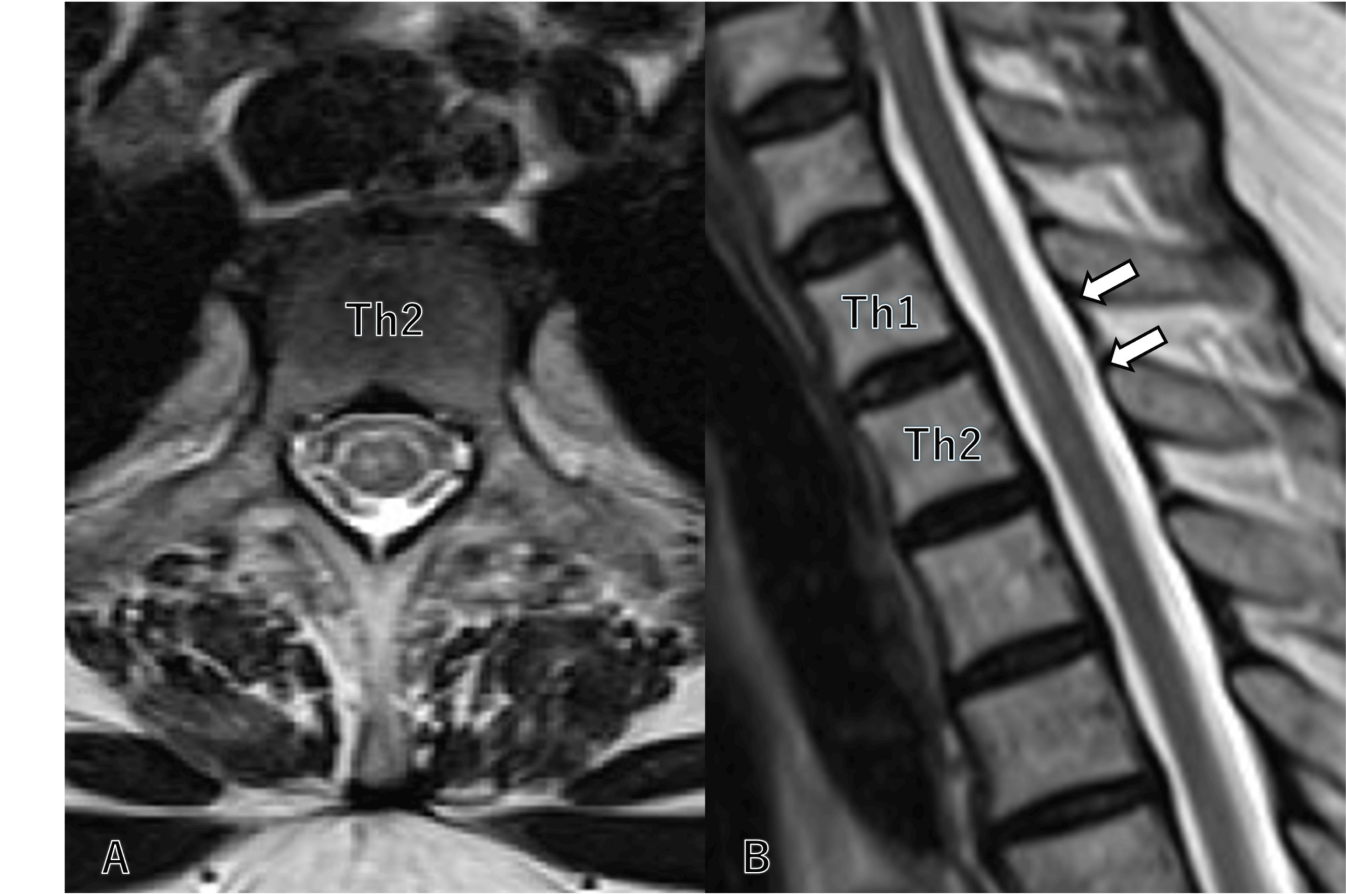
MRI performed 10 hours after symptom onset T2-weighted images show high signal intensity and diffusion restriction in the anterior horns (A) of the spinal cord at the T1-T2 vertebral level (B).
